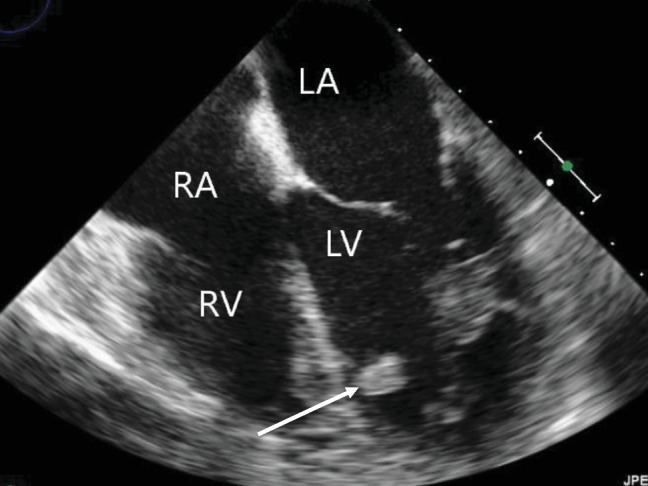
Transesophageal echocardiograph showing rupture mitral papillary muscle (arrow) in relation to the right atrium (RA), left atrium (LA), right ventricle (RV), and left ventricle (LV). TEE was obtained due to the presence of pulmonary edema with new holosystolic murmur on day 3 s/p MVC.
